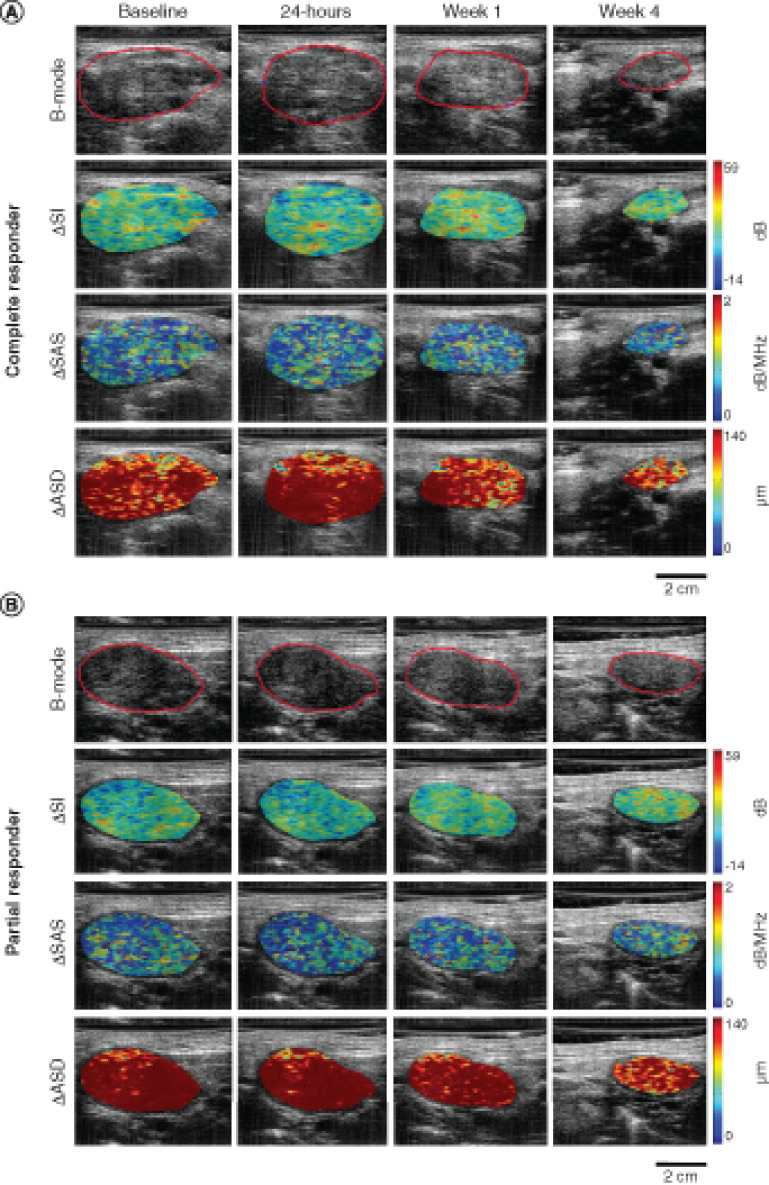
Quantitative ultrasound parametric maps. Representative QUS parametric image overlays of ΔSI, ΔSAS and ΔASD at baseline, 24 h, week 1 and 4 of treatment for a complete responder (A) and a partial responder (B). The ultrasound B-mode images have been contoured to delineate the lymph node that was scanned. ASD: Average scatterer diameter; QUS: Quantitative ultrasound; SAS: Spacing among scatterers; SI: Spectral intercept.
